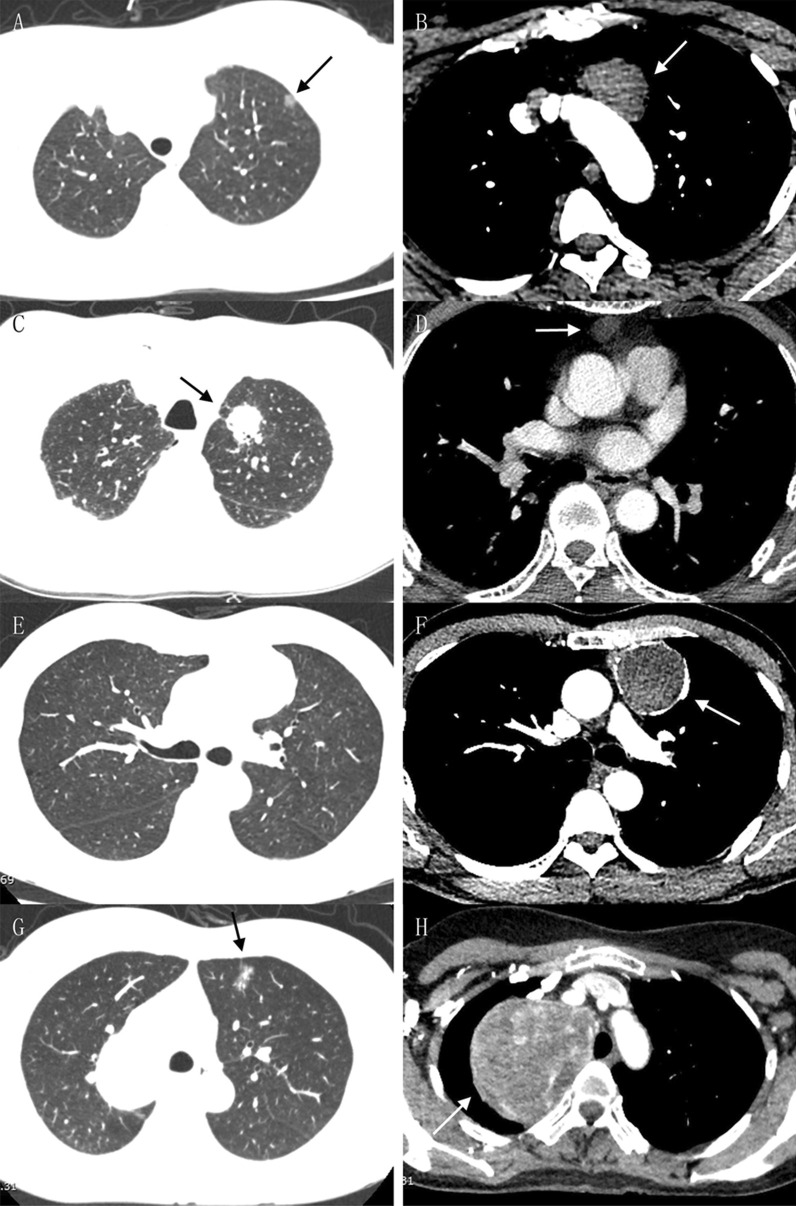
Chest CT images of representative patients. The arrows denote lesions. A, B A 10 mm left upper lobe GGO (microinvasive adenocarcinoma) associated with a 38 mm anterior mediastinal mass (hemangioma). C, D A 35 mm left upper lobe mass (invasive adenocarcinoma) associated with a 13 mm anterior mediastinal mass (bronchogenic cyst). E, F A 50 mm anterior mediastinal lesion (thymoma of type B2) invading surrounding lung tissues. G, H A 70 mm mediastinal mass (thyroid follicular neoplasm) and its coexisting 15 mm left upper lobe lesion (microinvasive adenocarcinoma), which were difficult for VATS and resected by thoracotomy
